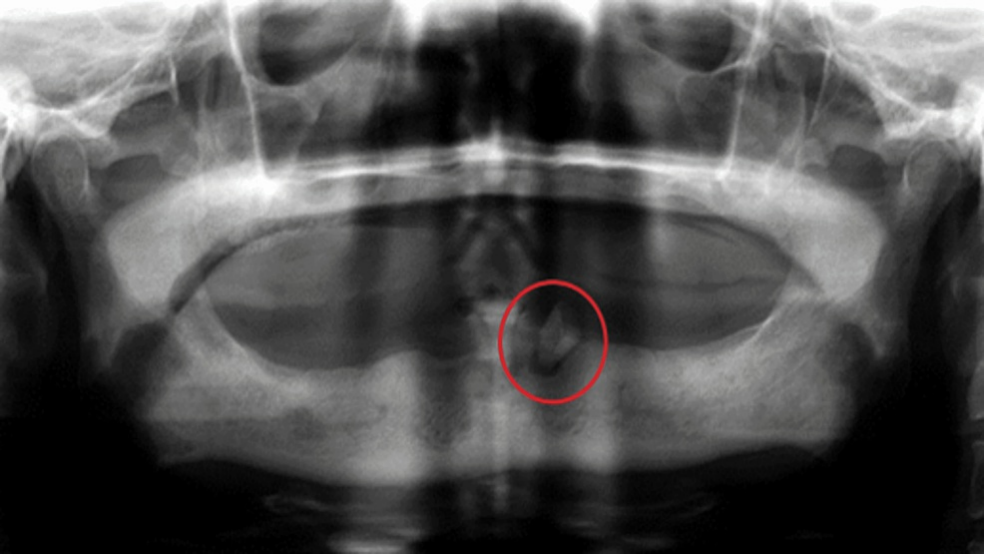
Retained root stump as seen on a panoramic radiograph
